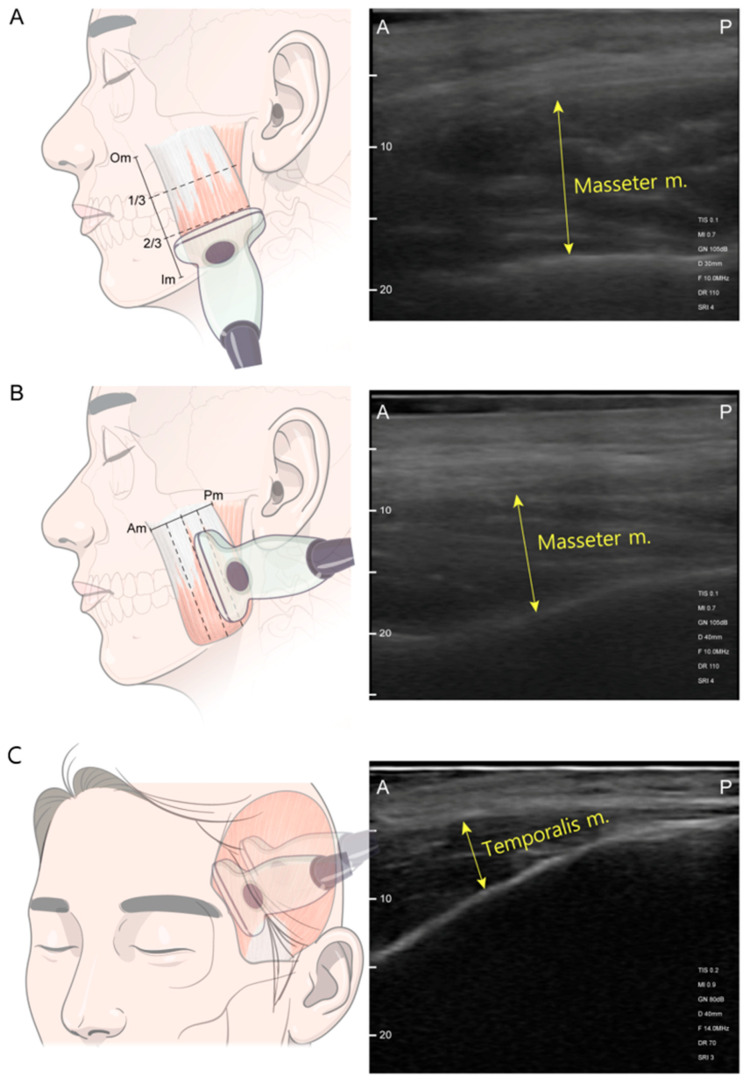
Ultrasonography scanning sites. (A) Transverse section scanning was performed at the upper-, mid-, and lower-third level between the masseter muscle origin (Om) and the masseter muscle insertion (Im) along the black dashed line. (B) Longitudinal section scanning was performed along the blue dashed line passing through the mesial, mid-, and distal points between the anterior border of the masseter muscle (Am) and the posterior border of the masseter muscle (Pm). (C) Temporalis muscle scanning at anterior and posterior points of the hairline. Abbreviations: 1/3, one-third level between the Om and Im; 2/3, lower-third level between the Om and Im; A, anterior; P, posterior.
